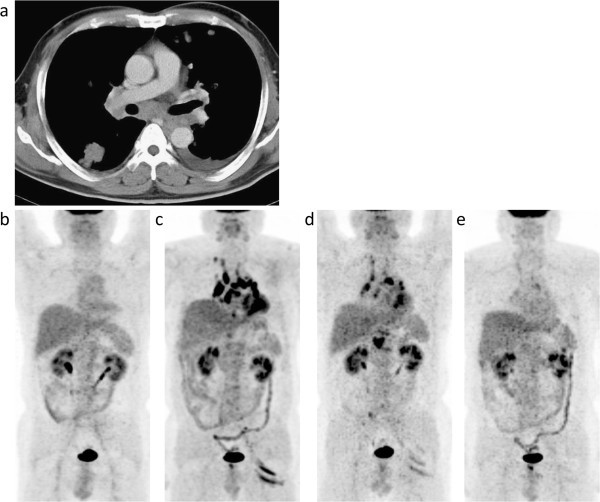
Case 1. A contrast-enhanced CT image taken at the time of the diagnosis of nonseminomatous malignant germ cell tumors with multiple intrathoracic and abdominal lymph nodes and lung metastases (a) and maximum intensity projection (MIP) images of FDG-PET/CT scans that were conducted 3 (b) and 6 years (c) after the completion of antineoplastic therapy and 8 (d) and 15 months (e) after the detection of FDG-avid lesions. (a) CT images before antineoplastic therapy showing metastases in the bilateral hilar and mediastinal nodes and lungs with pleural effusion. (b) No abnormal FDG uptake is observed. (c) Increased FDG uptake is evident in the bilateral hilar and mediastinal nodes (maximum standardized uptake value (SUVmax) 17.7), abdominal nodes, and the left gluteal muscles. (d, e) A gradual decrease to normal is observed concerning FDG uptake in these lesions.
